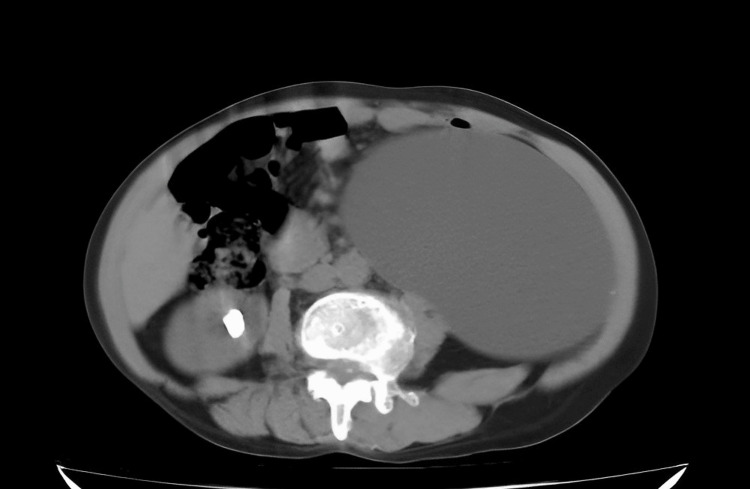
The CT scan image showed the left kidney with hydronephrosis and thin, dilated parenchyma.
